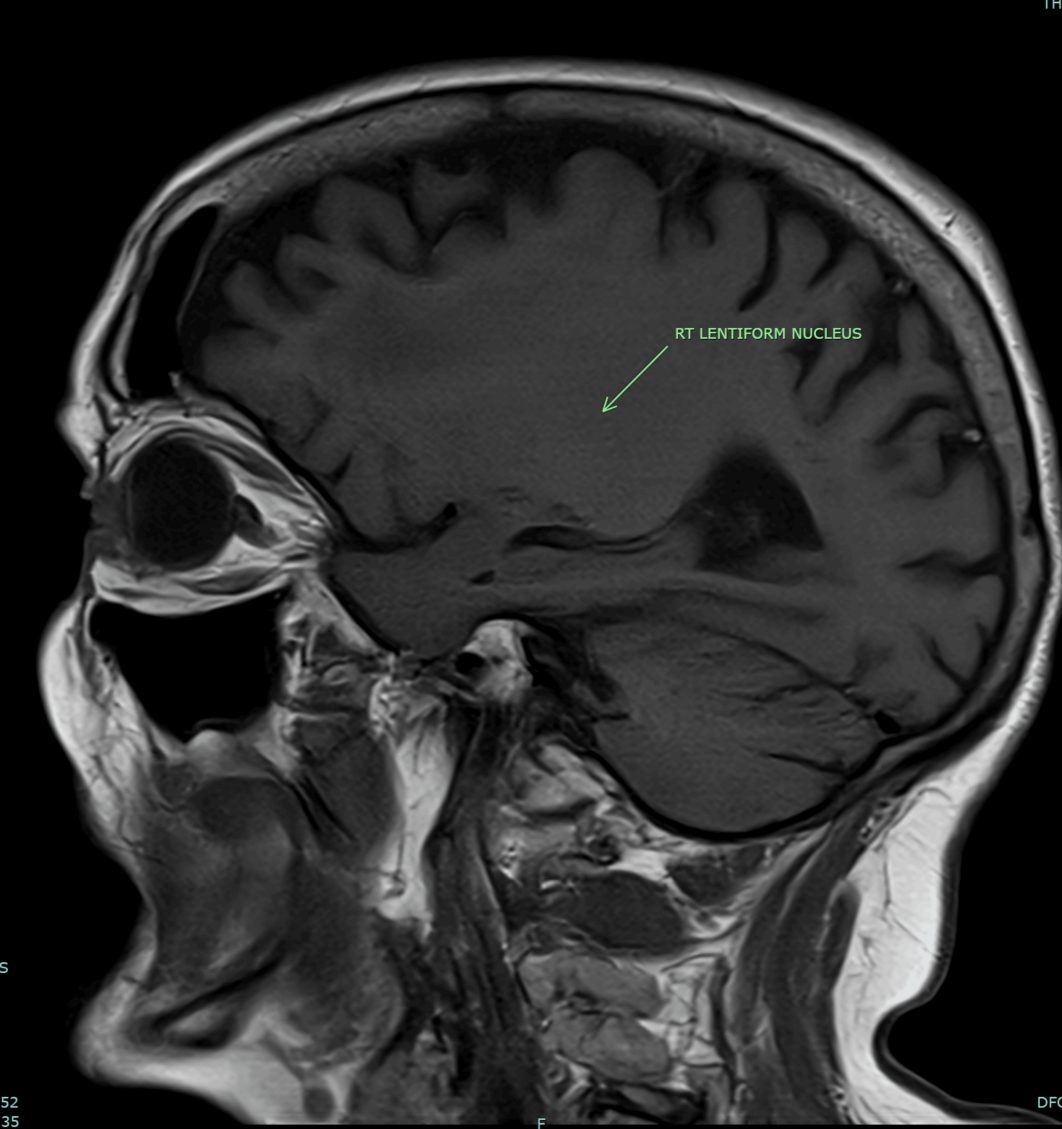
MRI brain T-1 parasagittal sequence image Area of the right lentiform nucleus is pointed out by the green arrow; no hyperintensity is noted.
